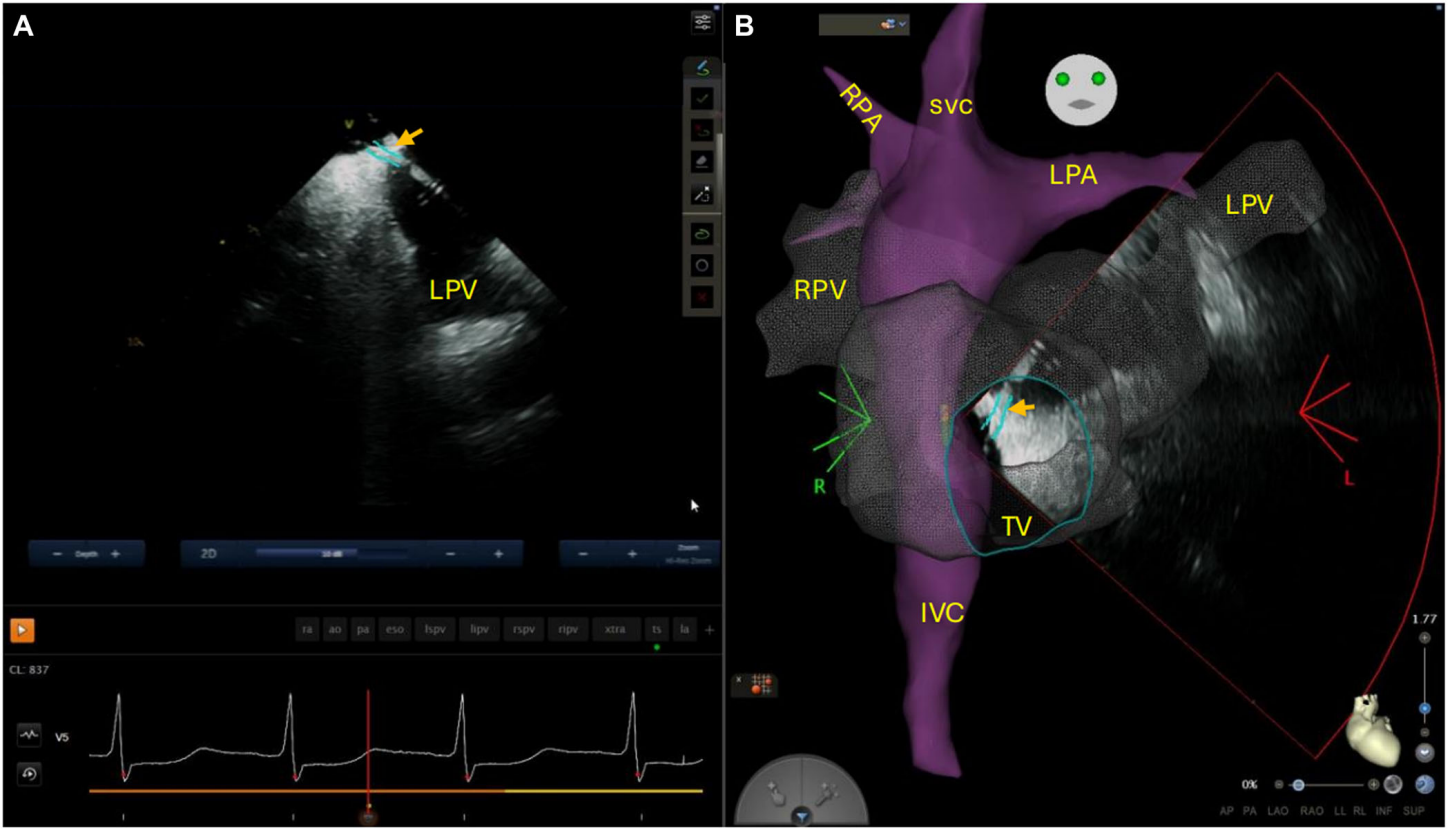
A transbaffle intracardiac echocardiogram (A) showing puncture site (orange arrow) with superimposition of a three‐dimensional electroanatomic map of the lateral tunnel Fontan (B). IVC, inferior vena cava; LPA, left pulmonary artery; LPV, left pulmonary vein; RPA, right pulmonary artery; RPV, right pulmonary vein; SVC, superior vena cava; TV, tricuspid valve.
